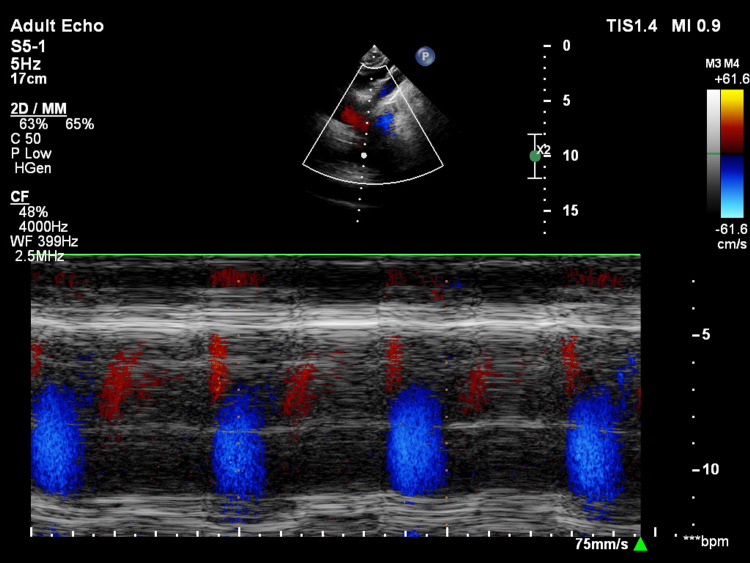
Color Doppler on motion-mode section across the descending aorta showing diastolic flow reversal.
